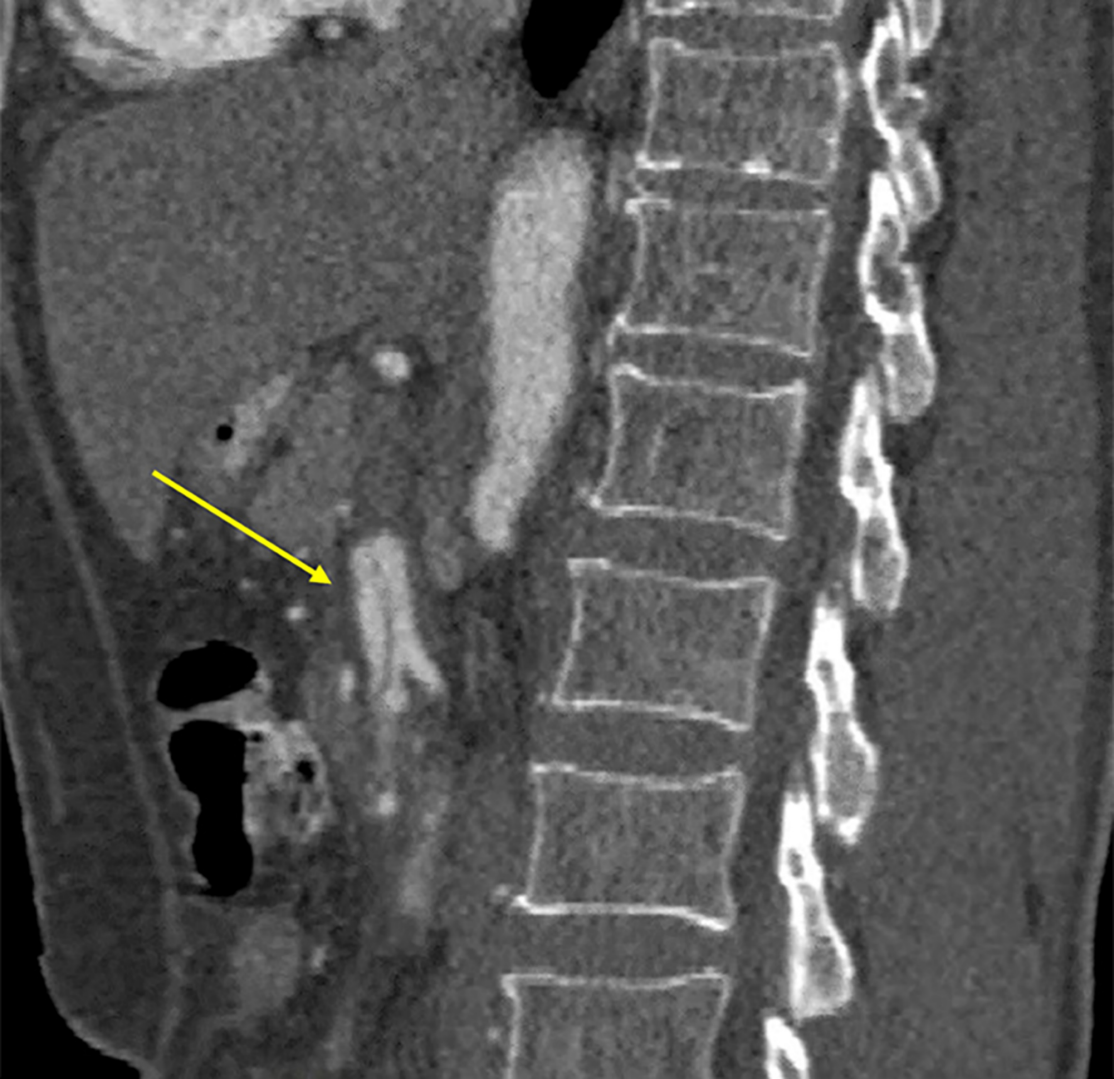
Sagittal reformatted CT aortogram image demonstrating dissection of the SMA. SMA, superior mesenteric artery
